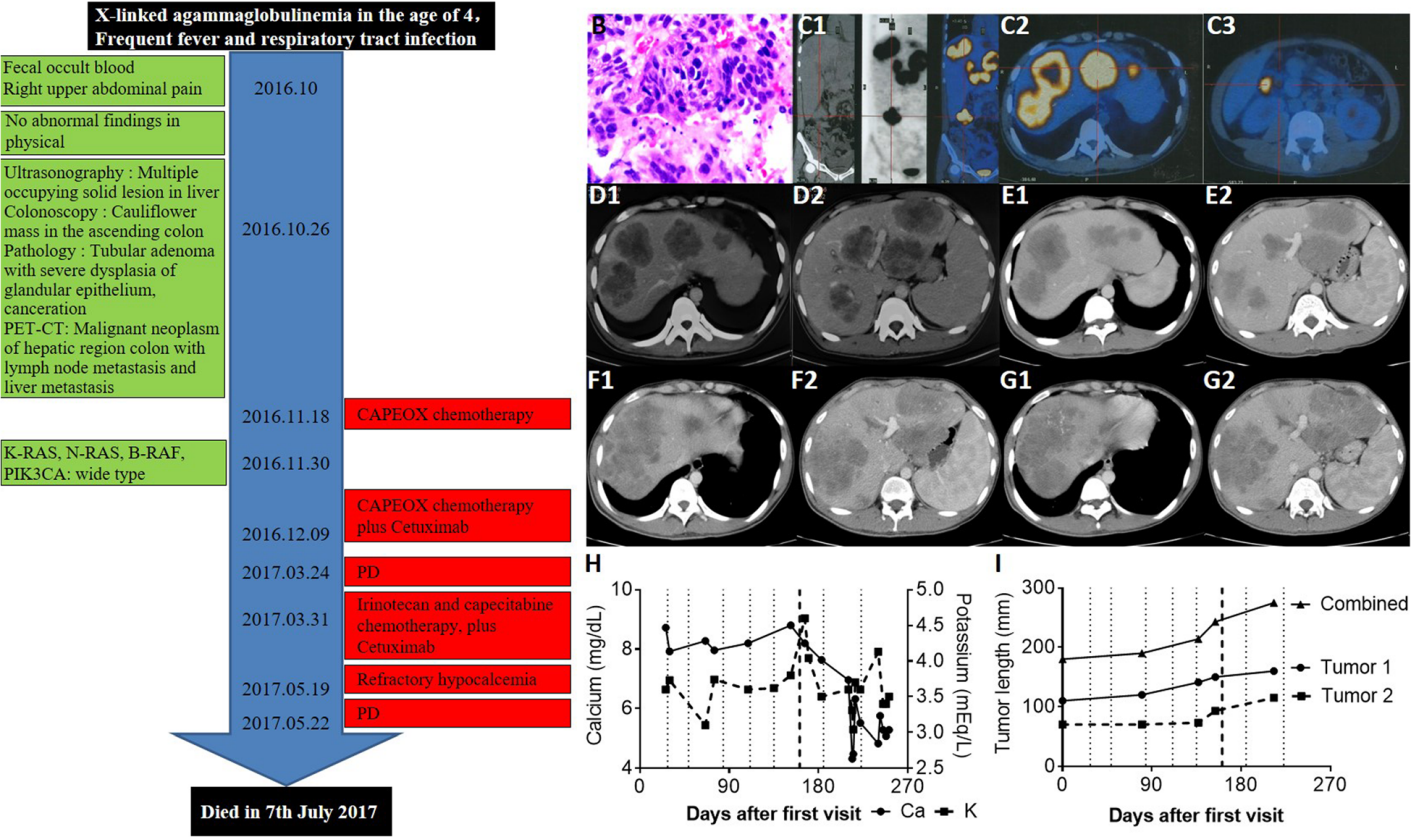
The timeline, diagnosis and progression focus. a The timeline of hospitalization: This patient was diagnosed with XLA at the age of 4. He suffered upper abdominal pain on Oct 2016, and was diagnosed with advanced colorectal cancer with liver metastasis by liver biopsy ((b), magnification, × 100) and PET-CT scan (c). Abdominal CT scans on Oct 21th 2016 (d) and Jan 9th 2017 (e) showed that the tumor was stabilized after the first two treatment cycles. However, abdominal CT scans on Mar 24th 2017 (f) and May 22th 2017 (g) showed metastatic tumor progressed. Blood calcium/potassium levels (h) and tumor load (i) were recorded throughout all treatment cycles. The dotted lines indicate the starting time of each chemotherapy treatment cycle. The dark dotted line indicates the sixth treatment cycle when oxaliplatin was replaced by irinotecan
